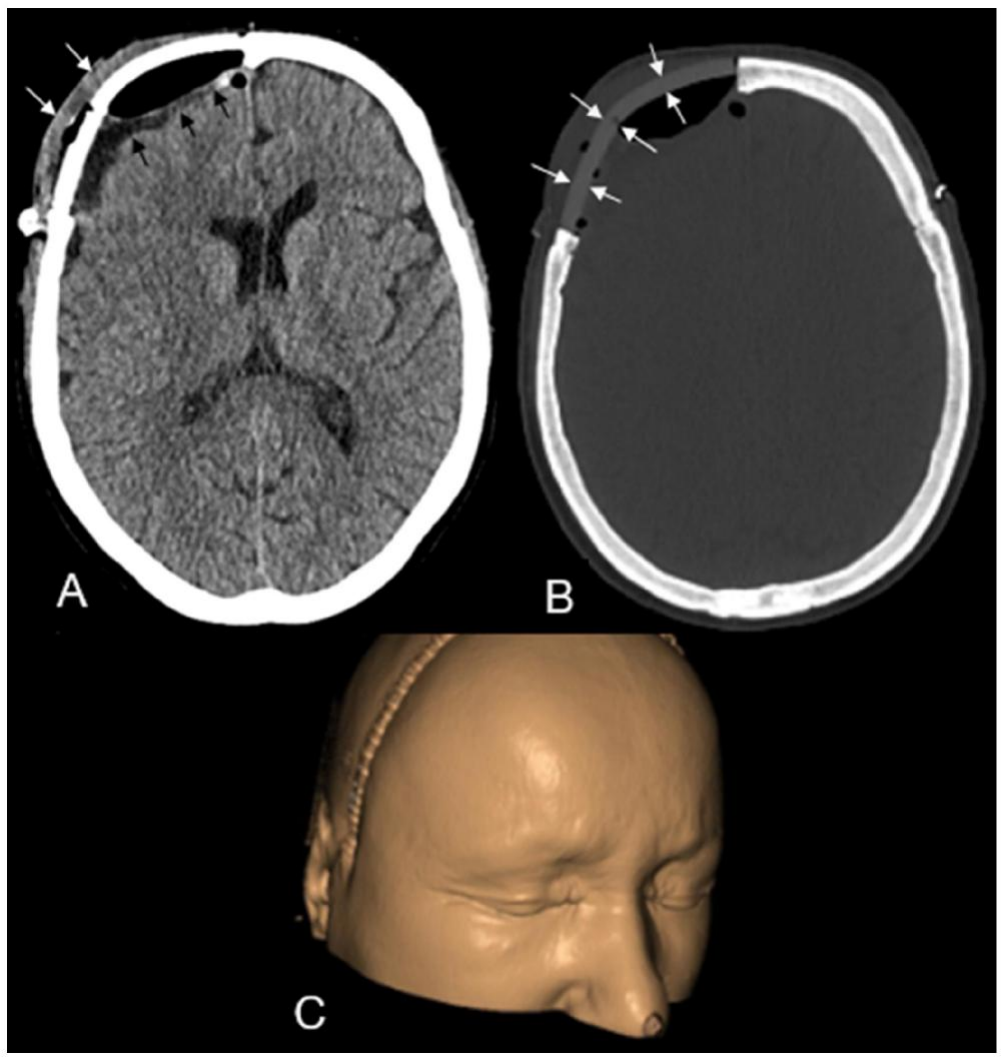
First postoperative computed tomography scan after macroscopic complete resection of the intra- and extracranial tumour components, along with the infiltrated frontal bone, with normal postoperative findings: including intracranial extraaxial gas inclusions and a small extraaxial hygroma with residual lavage fluid (black arrows in A) and mild extracranial soft tissue swelling (arrows in A). B shows the patient-specific implant used to cover the frontal bone defect. Figure 3C shows the 3D-assisted cranioplasty with the skin covered.
